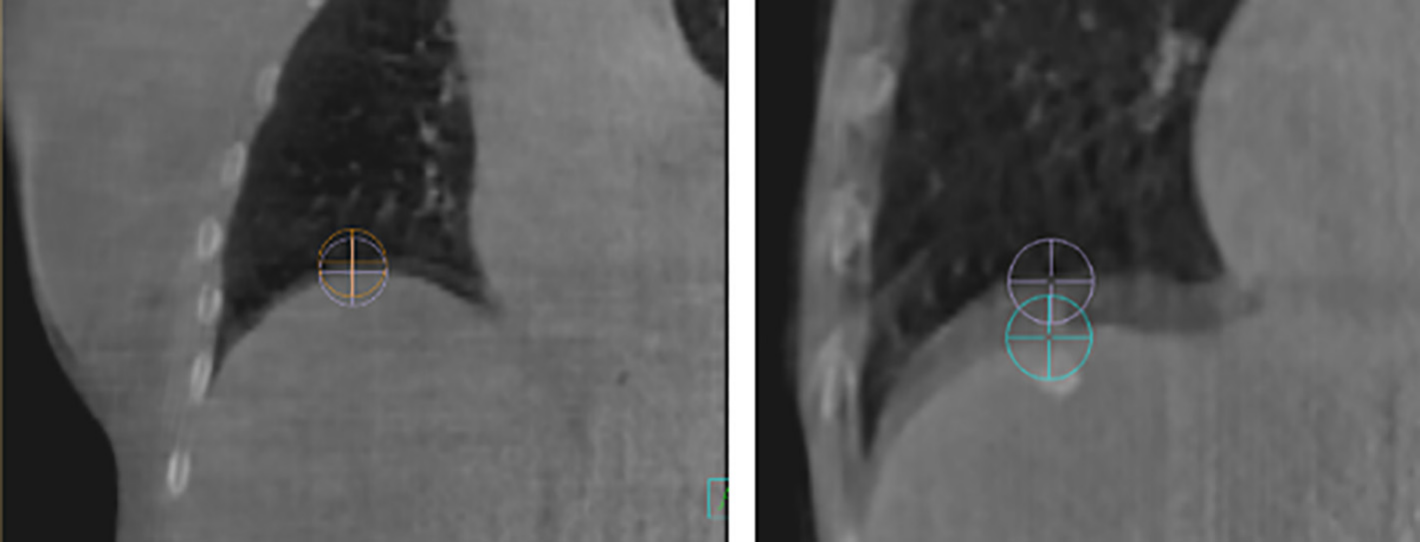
Example of BH‐induced motion blur artefact on the IF‐CBCTs of two different patients. The purple and blue points demonstrate the most superior and inferior visible edges of the diaphragm
